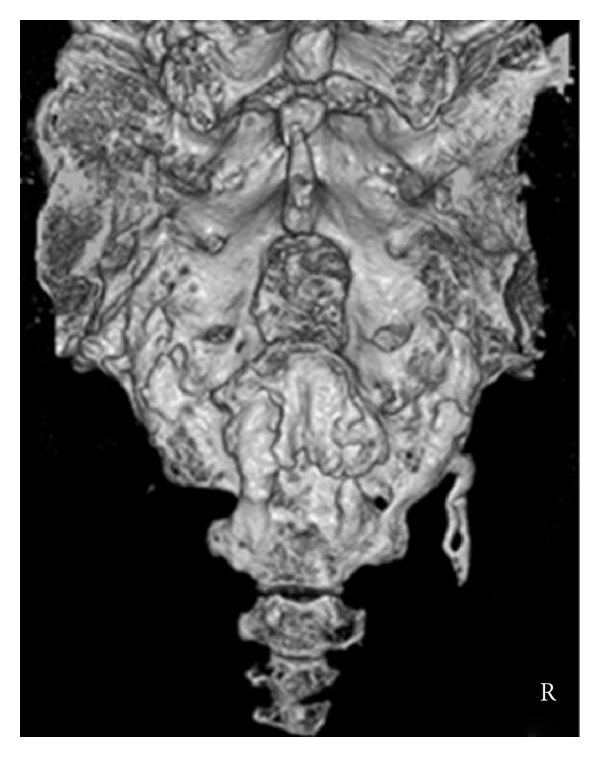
One-year postoperative follow-up three-dimensional CT Laminectomy remained and fracture lines in zone II disappeared.
